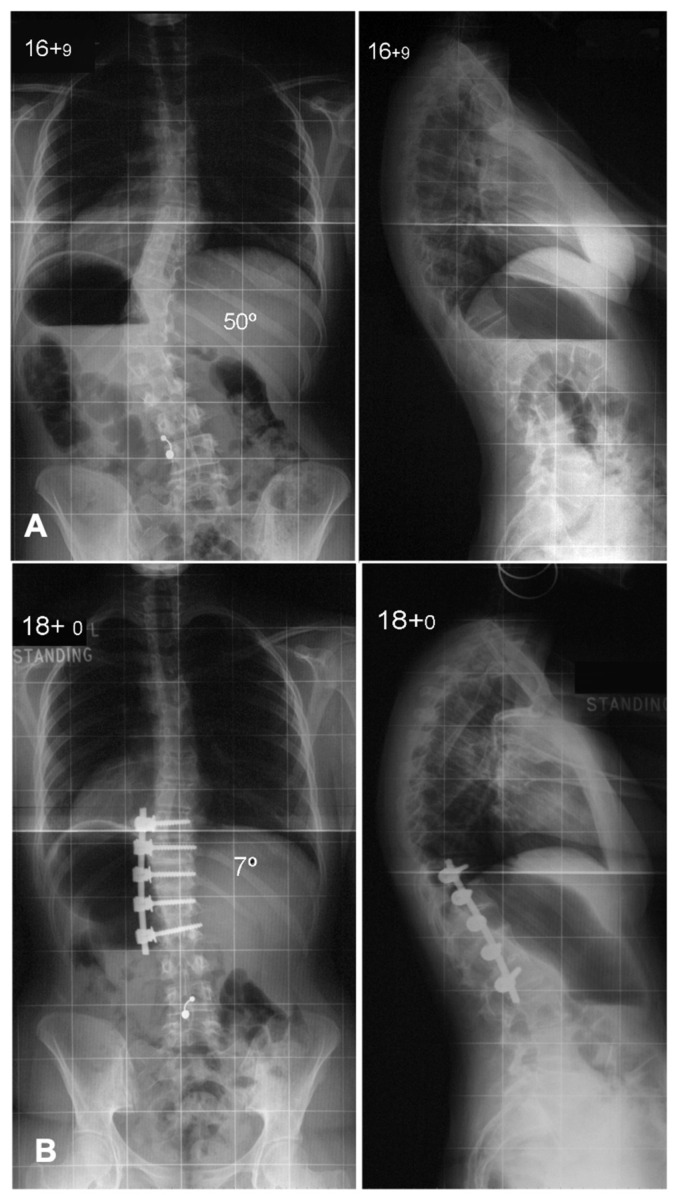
Deformity correction using anterior spinal fusion in a Lenke 5 curve. (A) Preoperative antero-posterior and lateral radiographs showing a thoraco-lumbar scoliosis with a Cobb angle of 50 degrees. (B) Postoperative radiographs showing good deformity correction with coronal and sagittal balance. Anterior spinal fusion allows good deformity correction with shorter fusion constructs.
